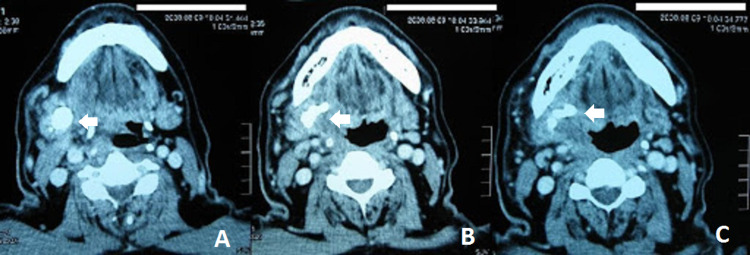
Axial images of the preoperative CT scan of the neck showing an opacity (white arrows) in the right submandibular gland and duct (A) A round opacity in the parenchyma of the right submandibular gland. (B,C) Medial extension of the sialolith to the proximal submandibular duct.
